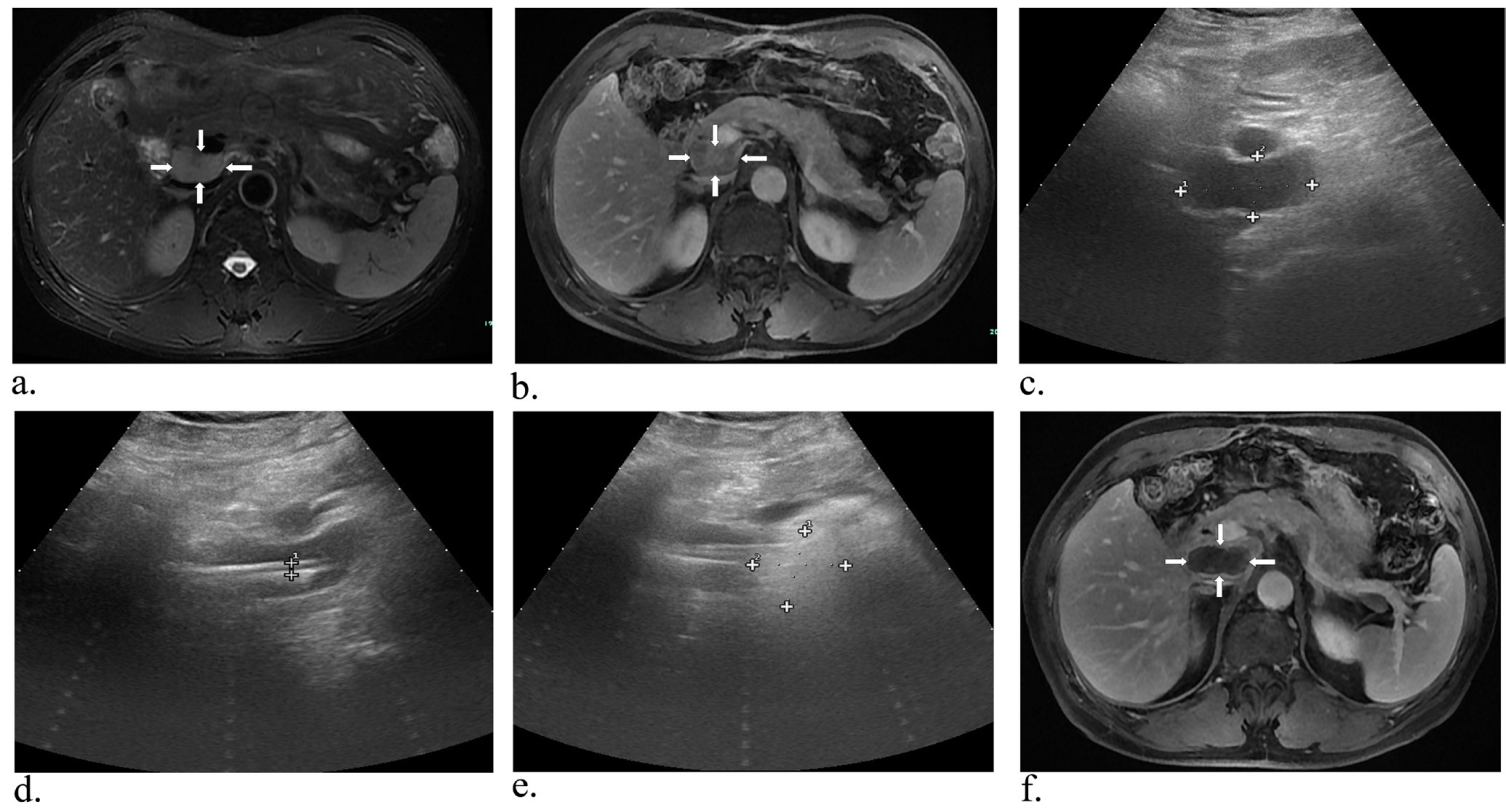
A 60-year-old man with metastatic lymph nodes beside the inferior vena cava Preoperative MR image in T2-weighted a. (white arrows) and substance phase b. (white arrows) suggested a mass of 3.9*2.1 cm in size close to the vena cava. Then with the guidance of US, two laser fibers parallelly were insered into and ablated the tumor c.-e. (white arrows). One month later, MRI scan indicated the lesion was complete necrosis f. (white arrows).
